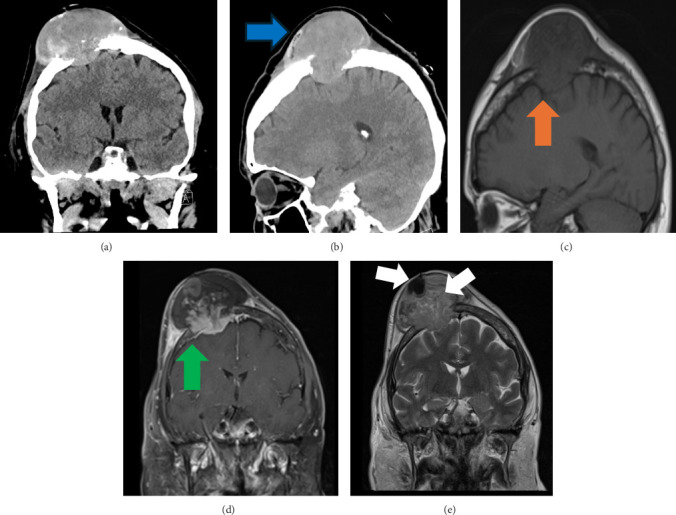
Computed tomography of the head. (A) Coronal view and (B) sagittal view. Brain magnetic resonance imaging: (C) sagittal T1 view, (D) coronal T1 view, and (E) coronal T2 view. Imaging showed a right paramedian parietal protruding mass (blue arrow) destroying the skull and extending to the epidural subdural space (green arrow) with localized mass effect (orange arrow) and internal calcification and necrosis (white arrow).
